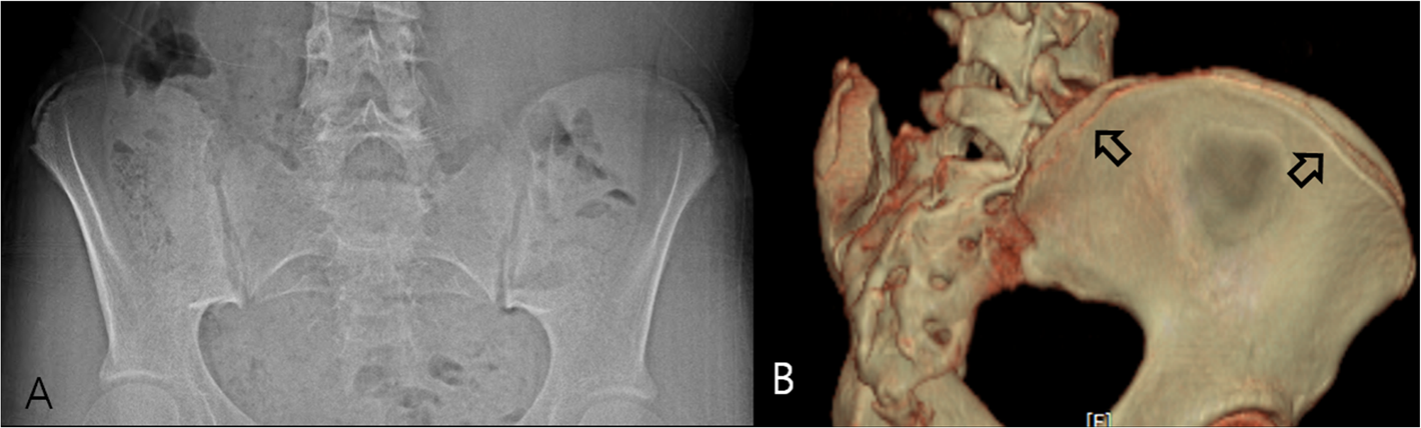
Skip ossification. (A) Plain radiography performed on a 14-year-old girl revealed excursion of the apophysis, approximately covering 60% of the iliac bone, and was graded as Original Risser stage 3 and French Risser stage 2. (B) A 3D-CT image of the same patient. Showing skipped ossification at the posterior iliac crest with grading of Original Risser stage 4 and French Risser stage 3. Initial apophyseal ossification of the ilium was not linear, but occurred in a skip pattern, which was not visualized properly by plain radiography.
